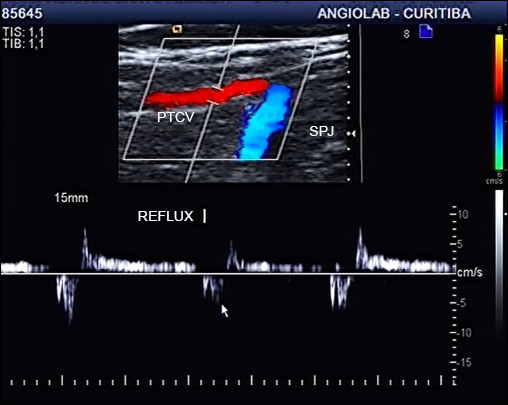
Ultrasound image of Giacomini vein with reflux. PTCV = Posterior thigh circumflex vein (Giacomimni Vein); SPJ = saphenopopliteal junction.
